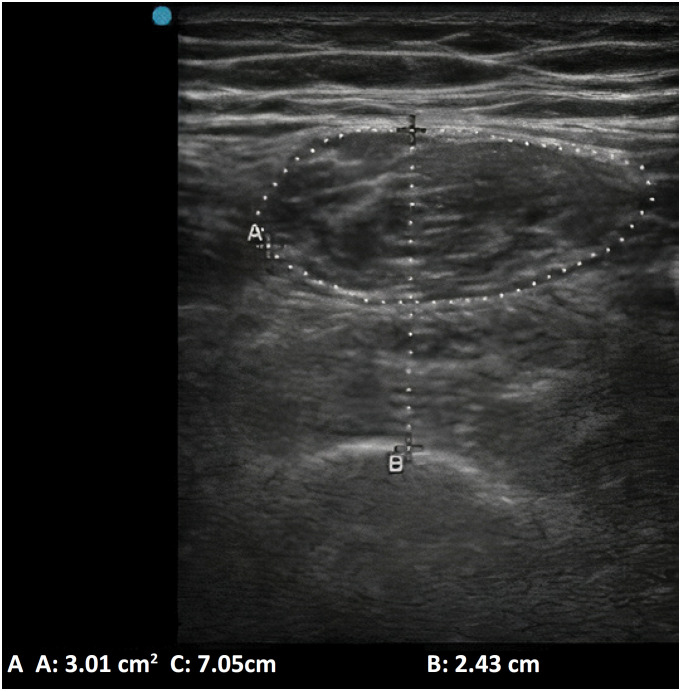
Ultrasound image acquisition
